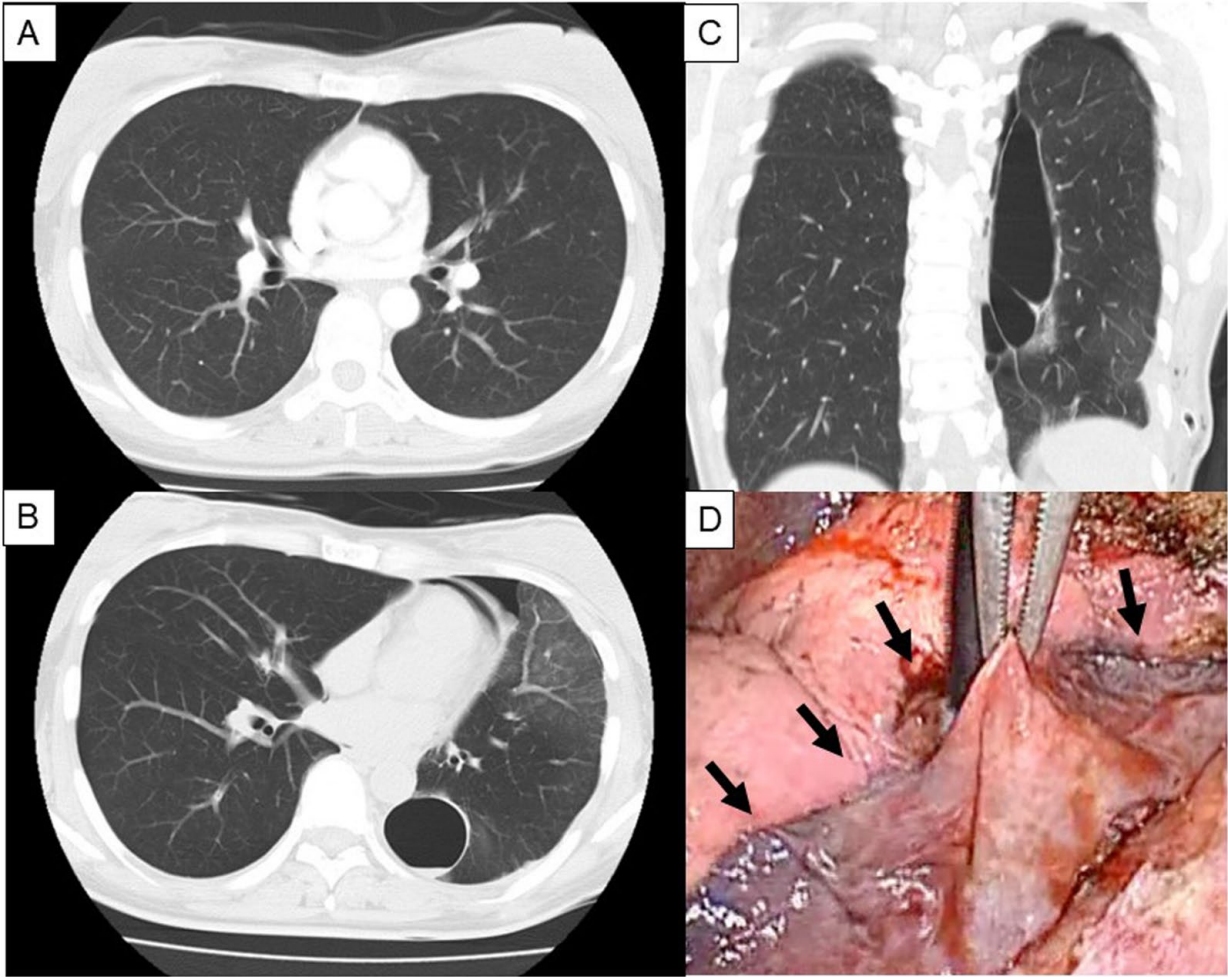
Preoperative CT and newly formed pulmonary cyst in Case 4. Preoperative CT shows a normal lung. (A). Postoperative CT performed 3 months after surgery reveals a newly developed pulmonary cyst at the posterior mediastinal surface of left upper lobe with ground glass opacity in left upper lobe (Axial section: B, Coronal section: C). Broad-based longitudinally huge pulmonary cyst with tear is recognized at re-operation (arrows) (D)
